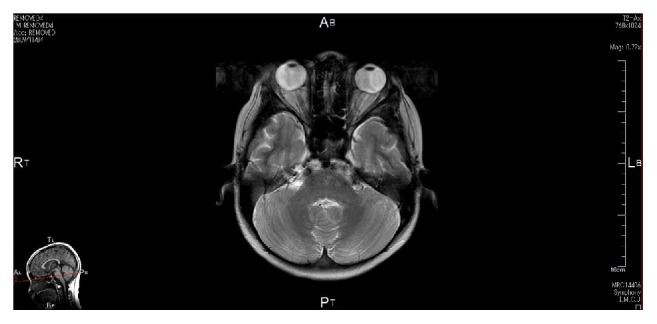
MRI findings of the patient with follicular lymphoma. MRI image shows thickening of the optic nerve sheaths causing “tram track” sign.
